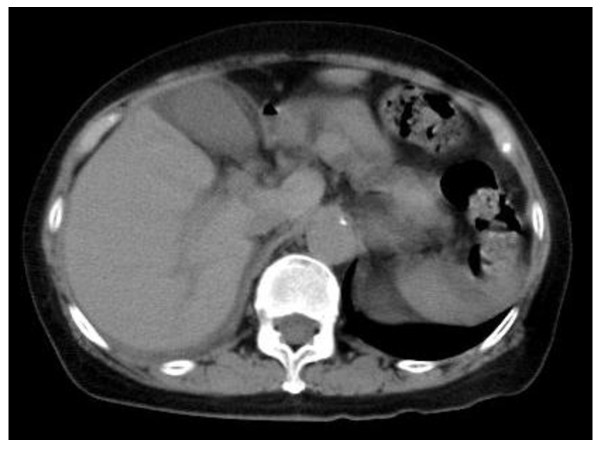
Abdominal CT showed a normal gallbladder at the first visit.
